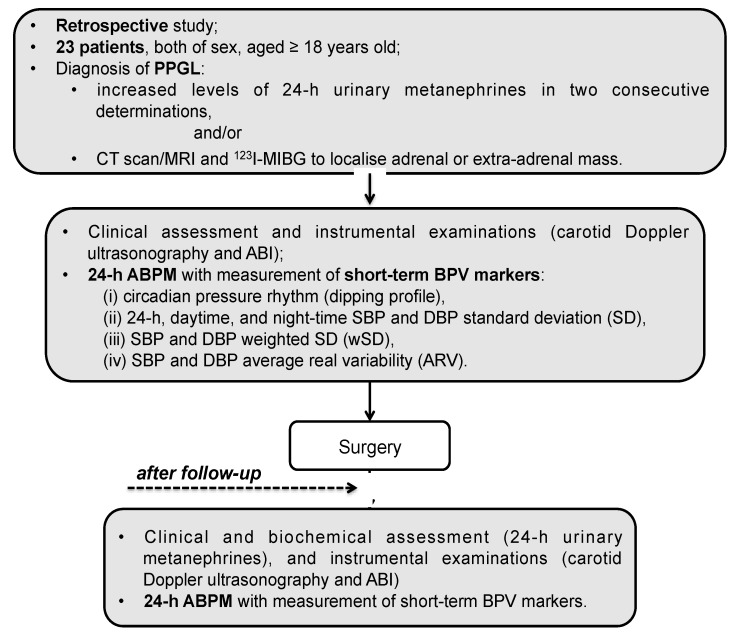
Flow-chart of the study. PPGL, pheochromocytoma and paraganglioma; CT, computed tomography; MRI, magnetic resonance imaging; MIBG, metaiodobenzylguanidine; ABI, ankle-brachial index; SBP, systolic blood pressure; DBP, diastolic blood pressure; SD, standard deviation.
